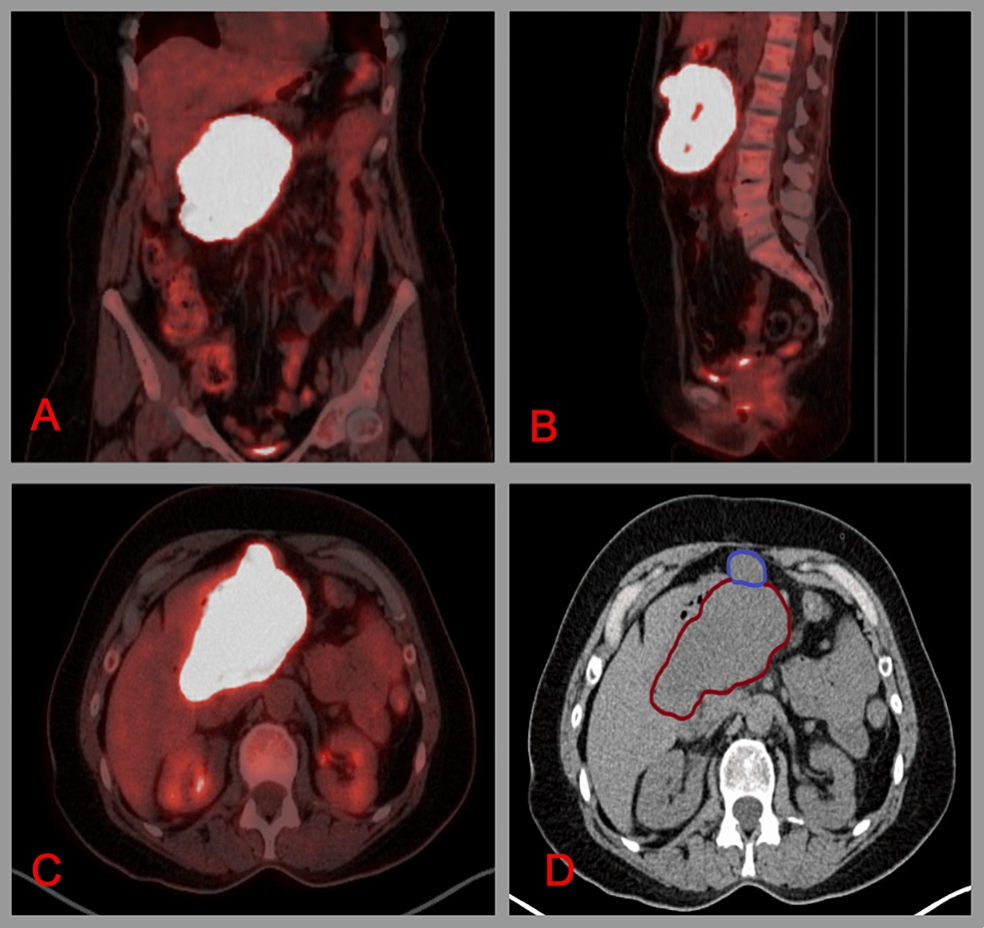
Positron Emission Tomography-Computed Tomography in Coronal (A), Sagittal (B), and Axial (C) Planes. Corresponding Computed Tomography of Abdomen with Red Outline Identifying Mesenteric Mass and Blue Outline Identifying Lymph Node (D).
